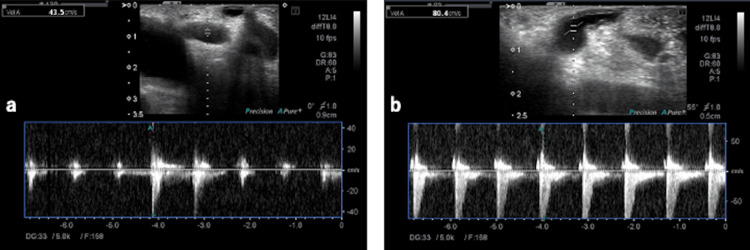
Intraoperative Doppler echocardiography. The figure shows a change in the mean flow velocity (43.5-80.4 cm/s) before and after MAL dissection, indicating that the blood flow is supplied with a more constant rhythm after dissection than that before dissection (MAL: median arcuate ligament)
